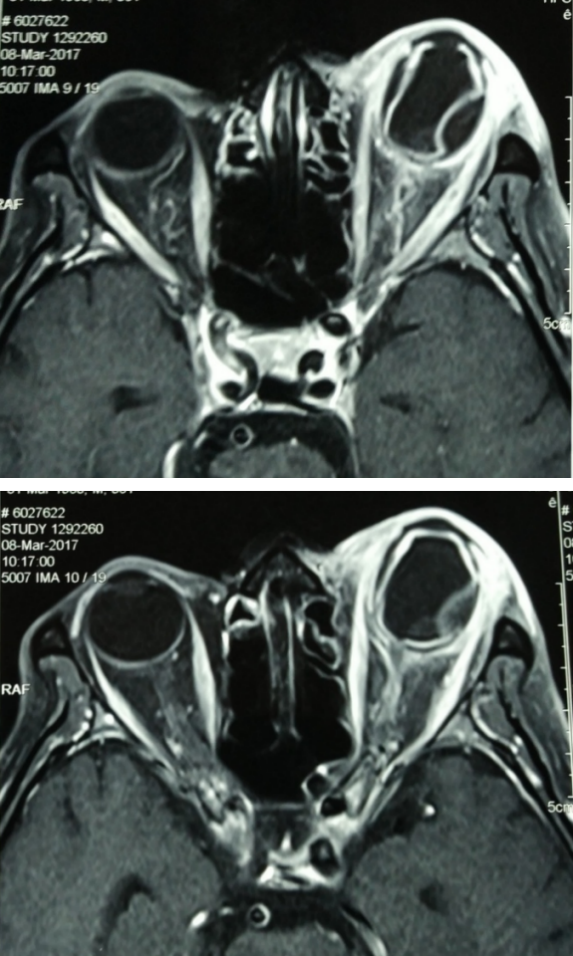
MRI orbit showing left choroidal detachment with lens displacement
